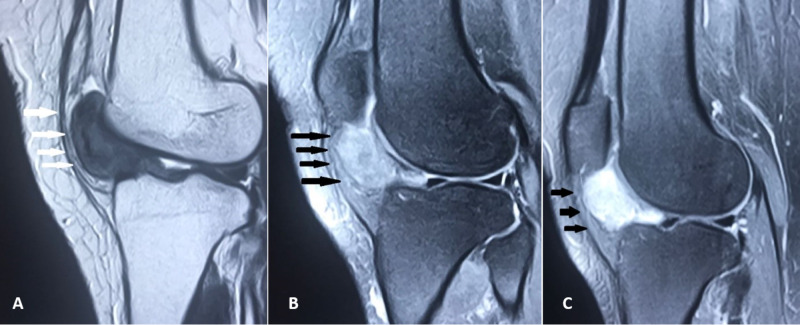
MRI of the left knee. MRI showing (A) isointensity to muscle (white arrows) in T1-weighted images, (B) low to intermediate signal intensity (black arrows) in T2 images, (C) post-contrast homogeneous enhancement (black arrows) in T1-weighted fat-suppressed images.
